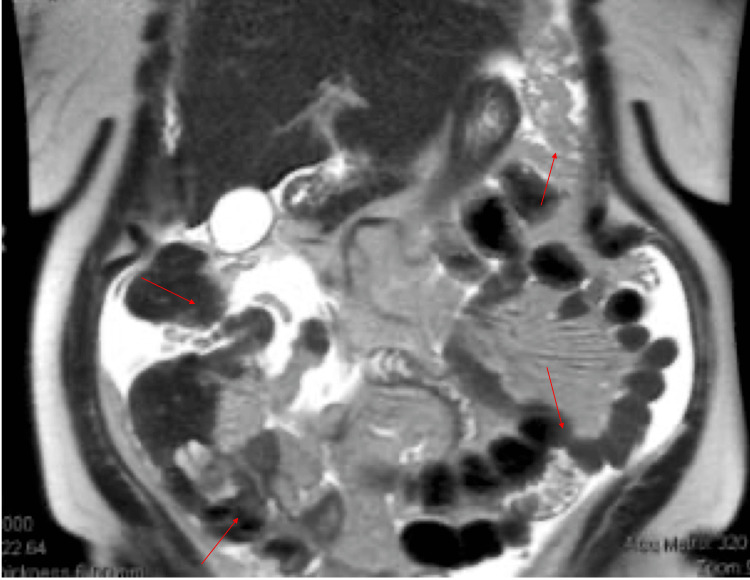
Abdominal T2-weighted coronal view image with contrast. Image demonstrating appendiceal and peritoneal thickening and nodularity concerning perforated appendicitis with peritonitis versus appendix malignancy with carcinomatosis (arrows).
